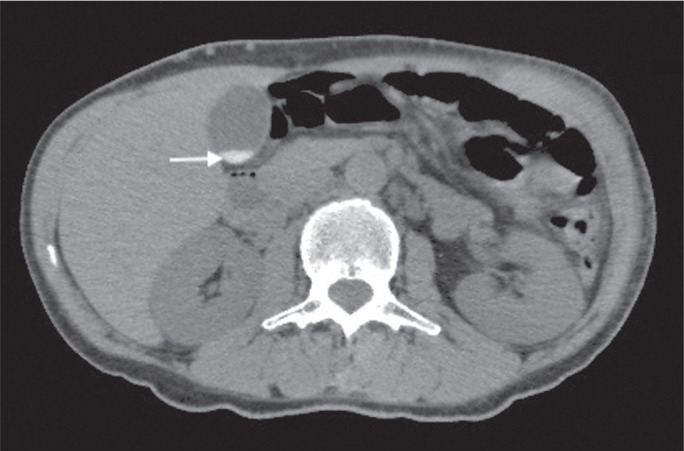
A 52-year-old male with dyspnea. Unenhanced axial CT image showing
